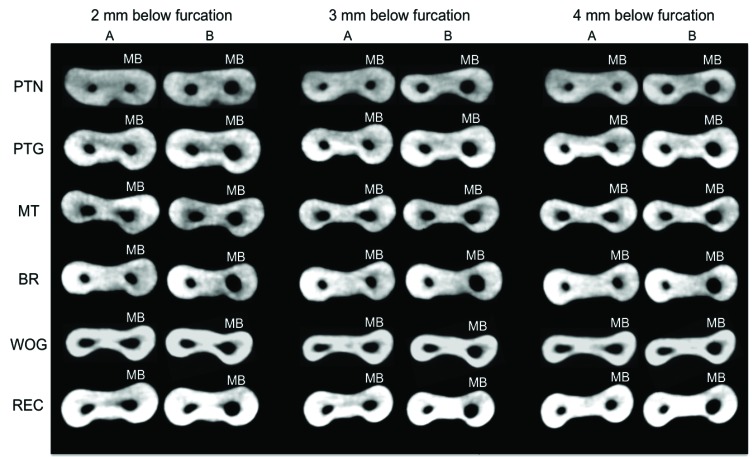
Cone-beam computed tomography scan (axial view) of cervical third of mesiobuccal root canals. A – before preparation. B – after preparation. PTN - ProTaper Next^®^; PTG - ProTaper Gold^®^; MT - Mtwo^®^; BR - BioRaCe^®^; WOG - WaveOne Gold^®^; REC - Reciproc^®^; MB – mesiobuccal canal.
